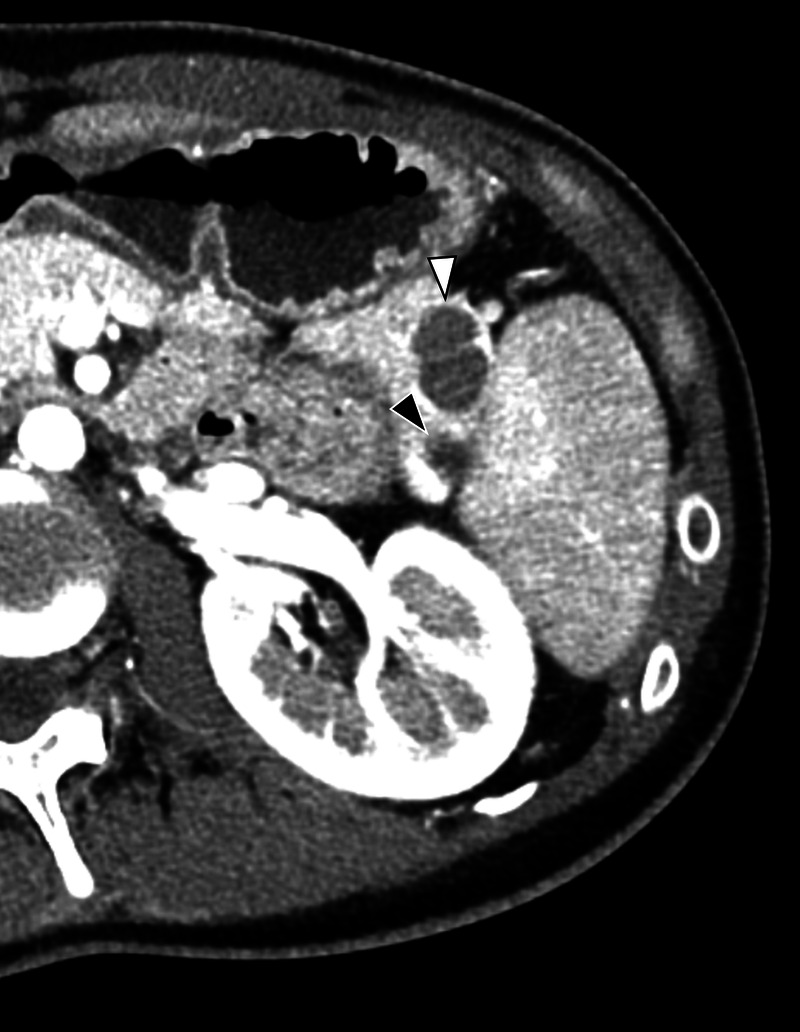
Contrast-enhanced computed tomography revealed a 17-mm multilocular cystic lesion (white arrow) and a 7-mm unilocular cystic lesion (black arrow) in the pancreatic tail. The margin of the cysts had the same enhancement as the spleen in the early arterial phase.
